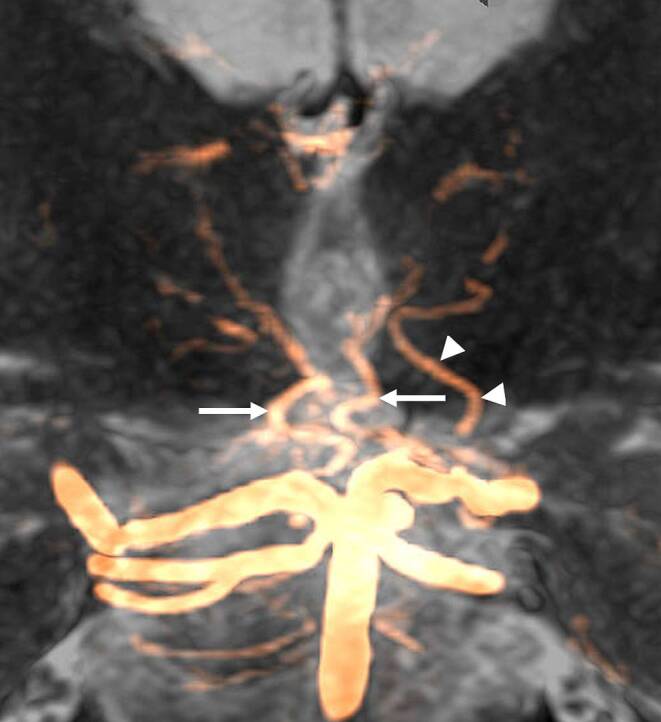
Coronal maximum intensity projection (MIP) of the posterior thalamoperforating arteries (PTA, arrows) and the left anterior thalamoperforating artery (ATA, arrowheads) projected onto a coronal T2 weighted MR image
